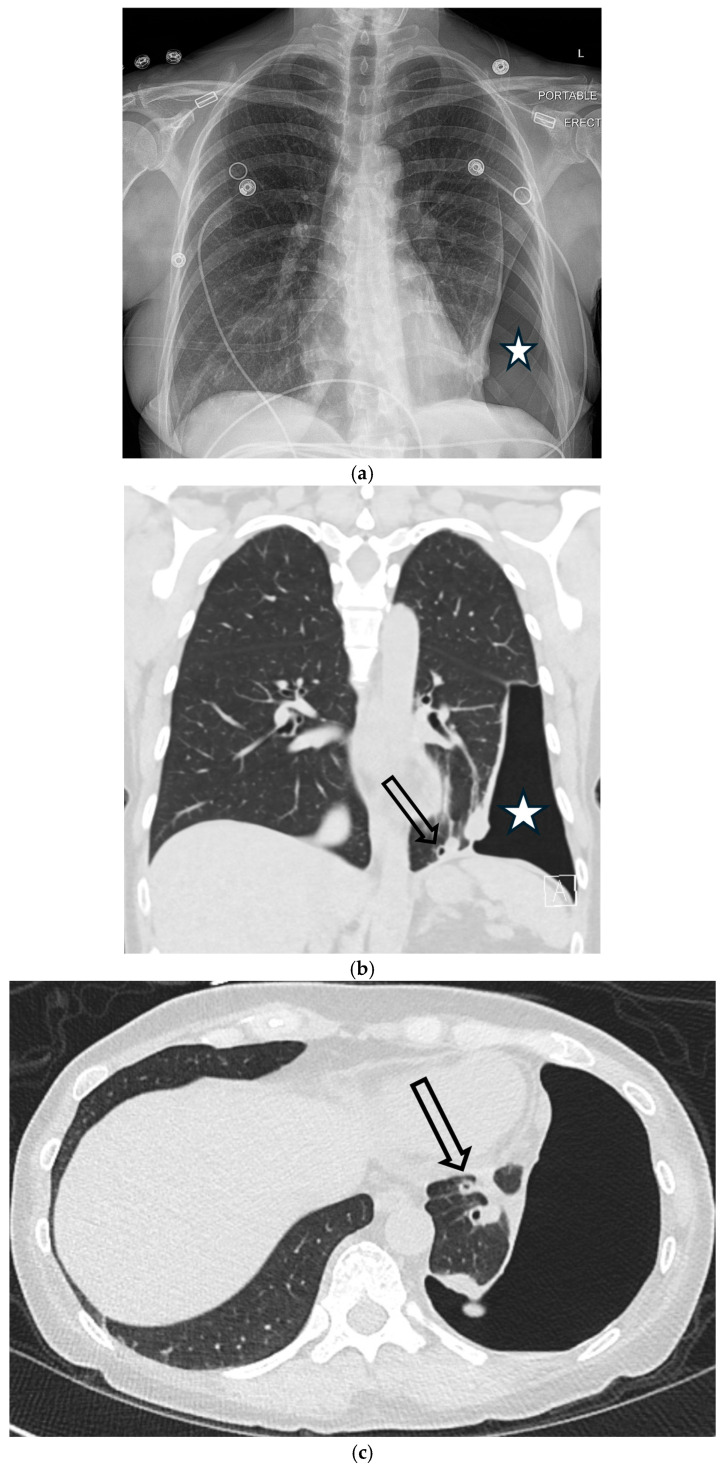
45-year-old female with rheumatoid arthritis presents with acute cough and chest pain. Frontal chest radiograph (a) demonstrates a large left basilar pneumothorax (white star) with partial atelectasis of the left lung. Coronal and axial CT images in lung window (b,c) again show left pneumothorax (white star), along with subpleural predominant solid and partially cavitary pulmonary nodules (open black arrow), in keeping with necrobiotic rheumatoid nodules.
